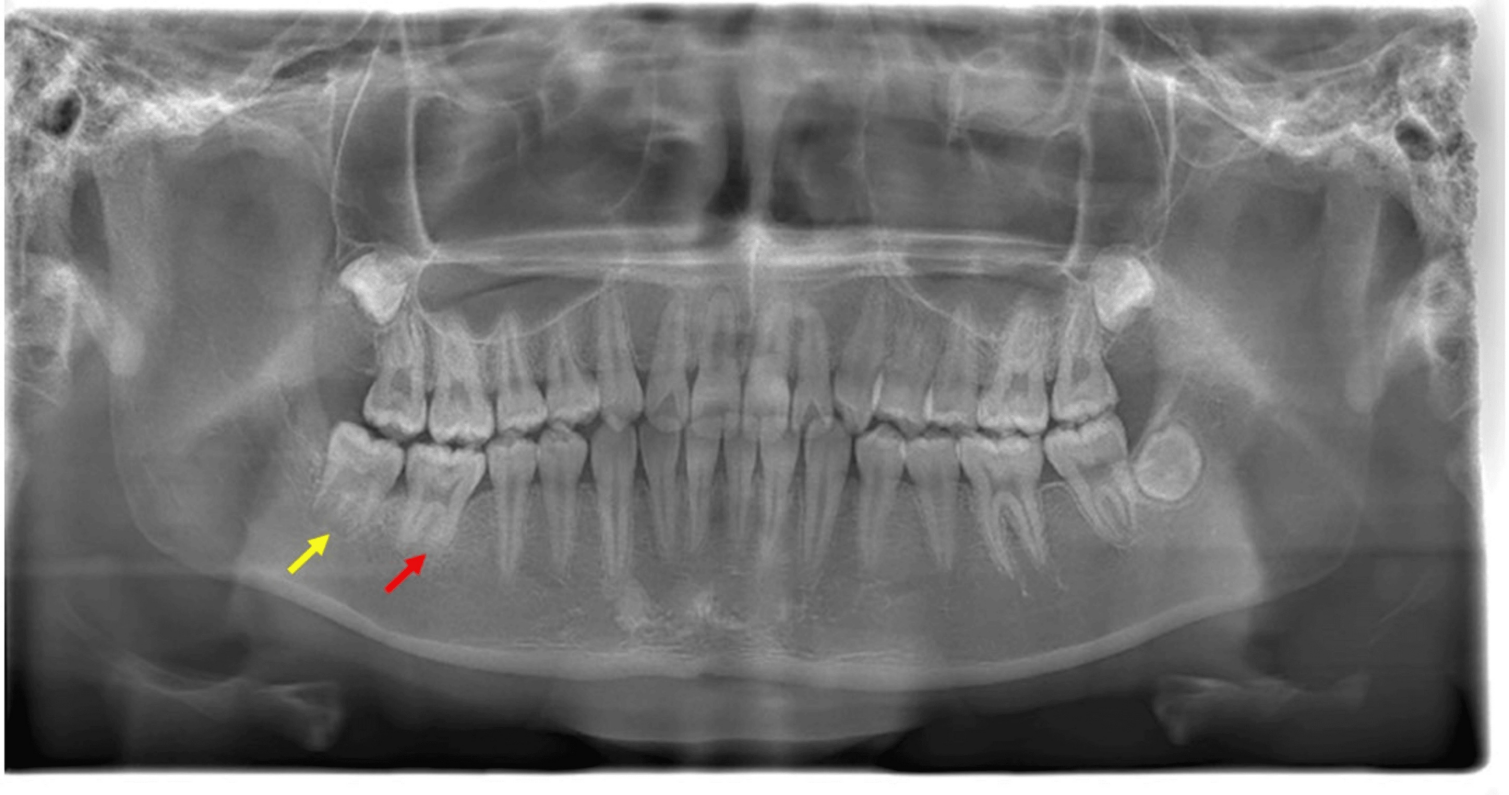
Postoperative panoramic radiograph of Case 2 A panoramic radiograph obtained four years postoperatively showing bone formation at the right mandibular area while the first molar demonstrated a normal direction of eruption (red arrow). The second molar apical closure was not obtained, however, a normal direction of eruption was shown (yellow arrow).
